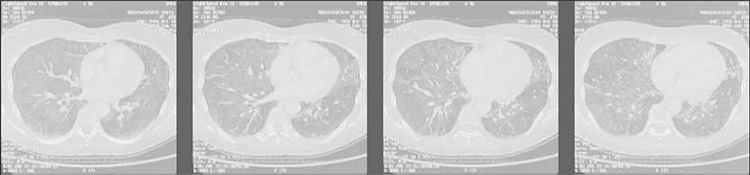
Chest CT: bilateral diffuse interstitial changes with fibrotic alterations in the lower parts of the lungs.
